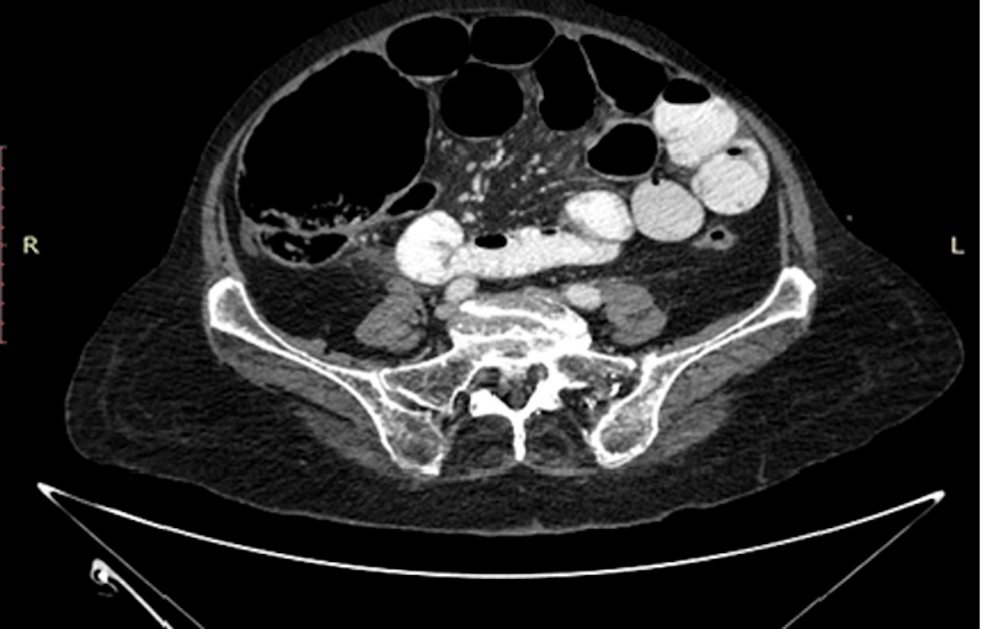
Representative CT image of the distended bowel as the result of obstruction
